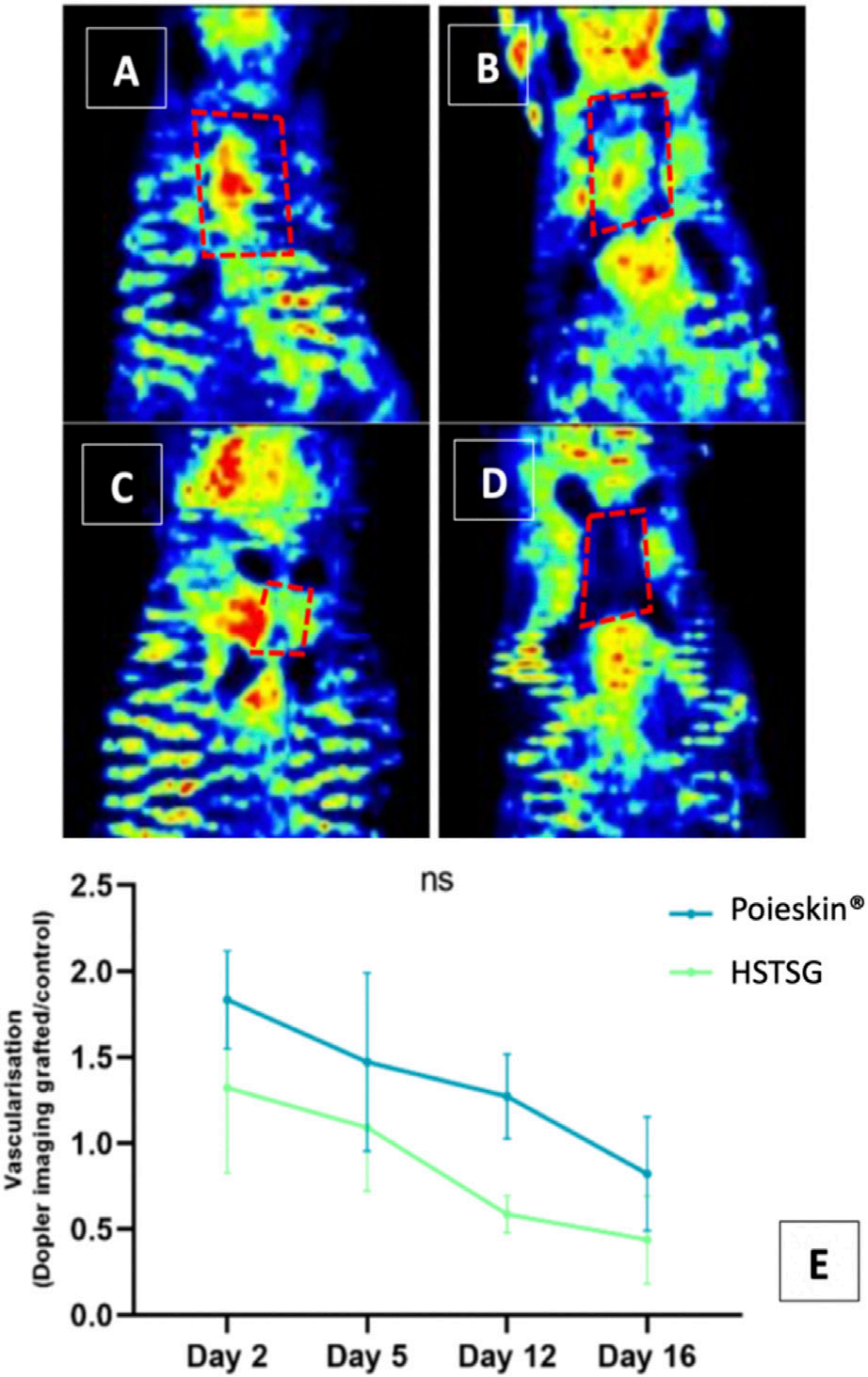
Graft perfusion assessment by laser Doppler ((A) Poieskin^®^ condition on day 2; (B) HSTSG condition on day 2; (C) Poieskin^®^ condition on day 16; (D) HSTSG condition on day 16; (E) evolution of the optical density ratio for both conditions).
